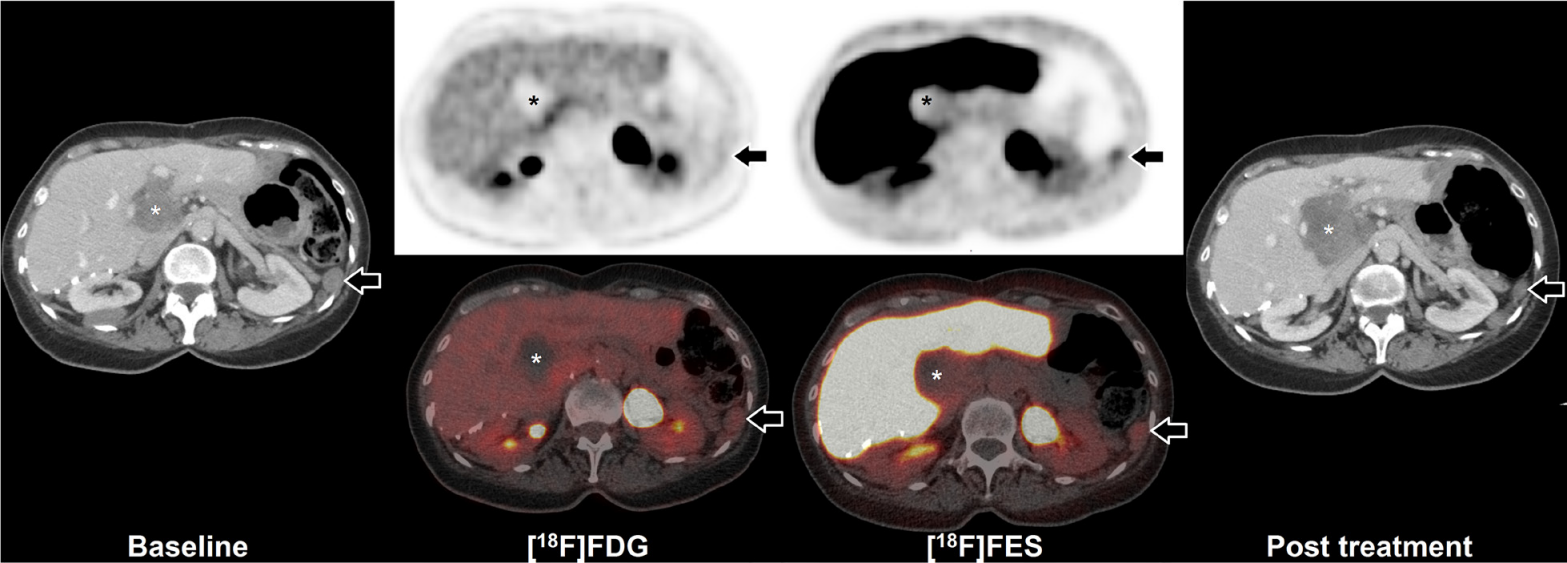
FES-PET and response to hormone treatment. Example of FDG and FES in a 64 year old woman with peritoneal and hepatic metastases of AGCT. Baseline CECT (left) shows peritoneal disease (arrow) and hepatic disease (asterisk). Additional FDG-PET/CT shows low uptake in the peritoneal disease, whilst FES shows moderate uptake (arrows). The majority of liver hilum lesion accumulates neither FDG nor FES (asterisks). After initiation of hormonal treatment with letrozole for six months, follow-up CECT (right) showed partial regression of the FES positive peritoneal lesion (from 23 mm to 17 mm maximal diameter; arrows), whilst the FES negative hepatic lesion showed progression (from 50 mm to 65 mm maximal diameter; asterisks).
